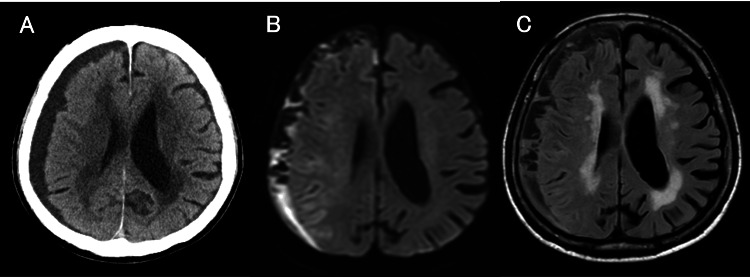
Imaging findings before endoscopic abscess removal in Case 3 A: Head CT reveals a slightly hypodense fluid collection in the right subdural space. B: Brain MRI-DWI revealed a mixture of high- and low-signal-intensity areas in the right subdural space along with a focal high-signal area on the brain surface, suggestive of a subdural abscess. C: FLAIR MRI reveals a mixture of hyperintense and hypointense areas suggestive of a pus-filled structure. CT, computed tomography; MRI, magnetic resonance imaging; DWI, diffusion-weighted imaging; FLAIR, fluid-attenuated inversion recovery
